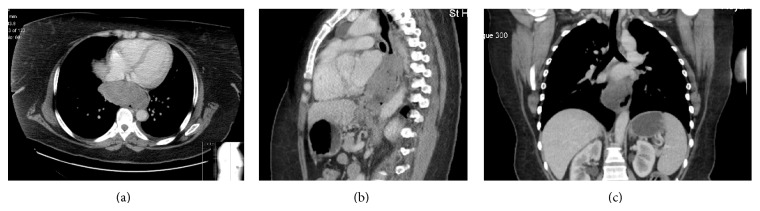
CT scan at presentation: (a) axial view, (b) sagittal view, and (c) coronal view.
